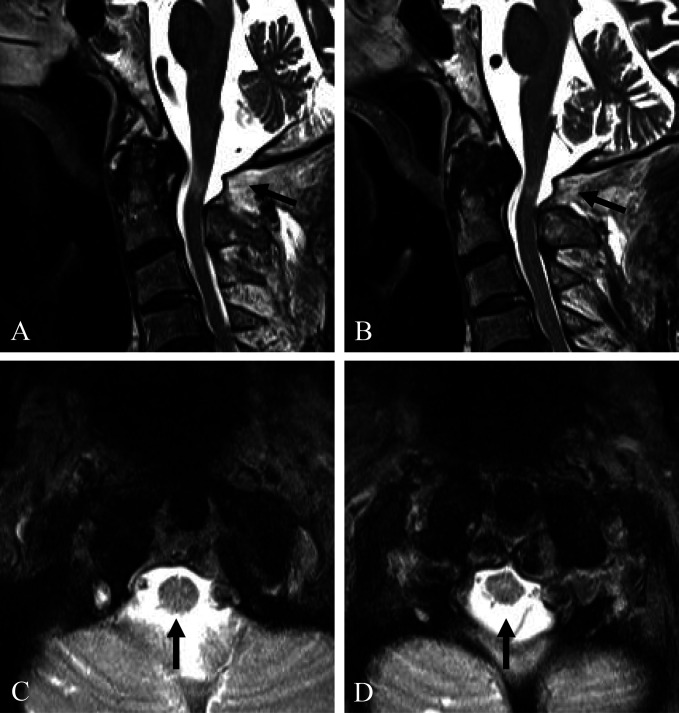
Postoperative MRI (A–D) showing decompression of the spinal cord after laminectomy of the C1 posterior arch (dark arrows).
